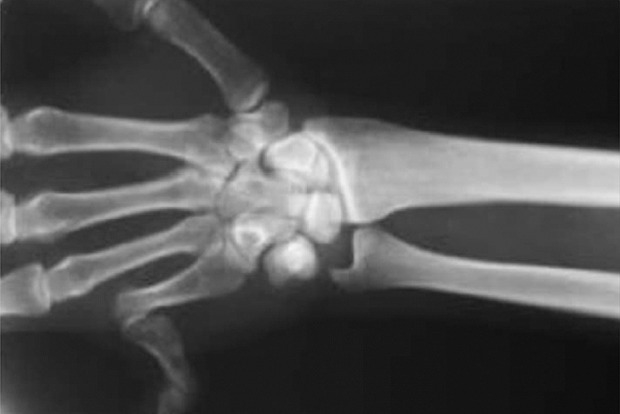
Radiograph of the small finger
